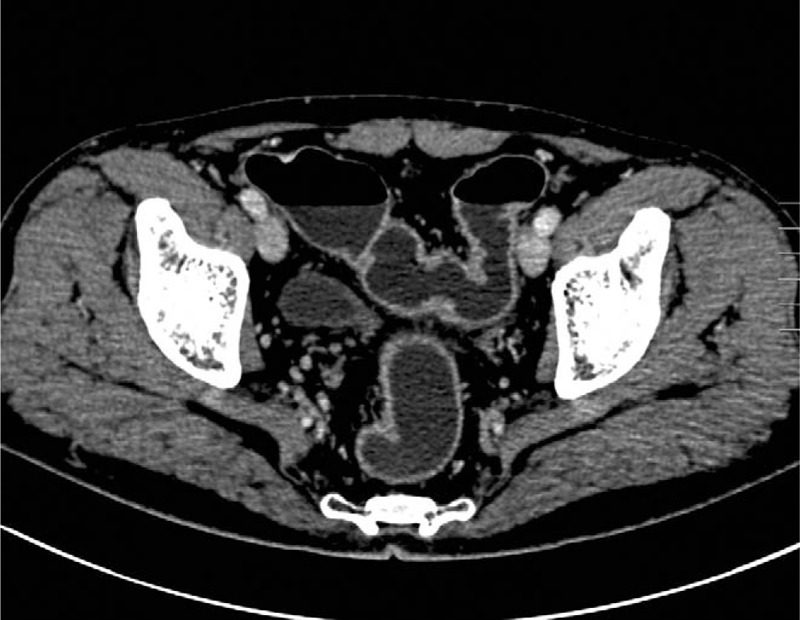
Abdominal CT image showed that the colonic masses resolved completely at the 1-month follow-up. CT = computed tomography.
